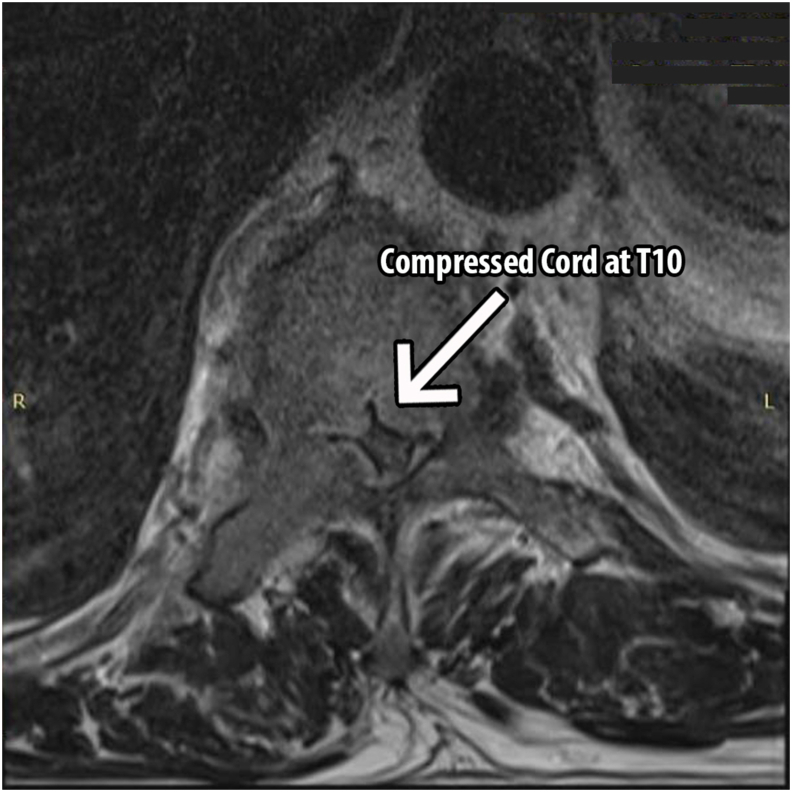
Axial view of the spine MRI.
